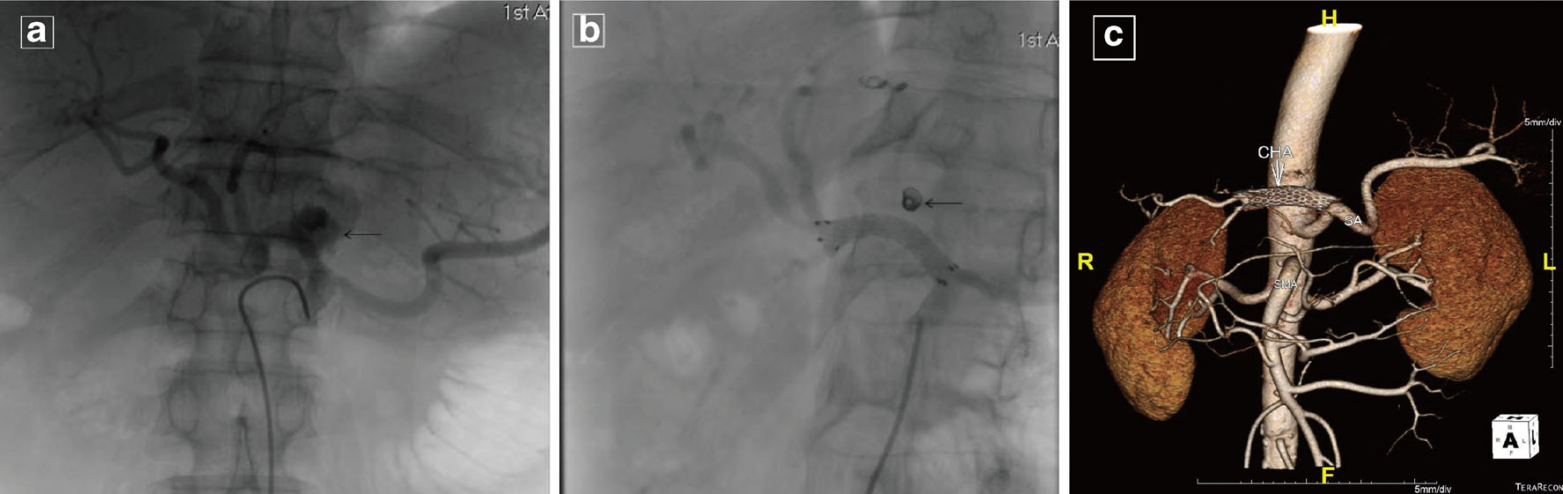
Bleeding pseudoaneurysm from the common hepatic artery. A 54-year-old man with distal gastrectomy for gastric cancer suddenly presented with severe abdominal pain at home, with a quick drop of hemoglobin 37 days after surgery. a Angiogram of the celiac axis shows a bleeding pseudoaneurysm (*arrow*) originating from the common hepatic artery. b The pseudoaneurysm was successfully planted with covered stent graft. The *arrow* shows the failed placing embolized coils. c The CT image reconstruction of abdominal arteries; *arrow* shows the covered stent graft
